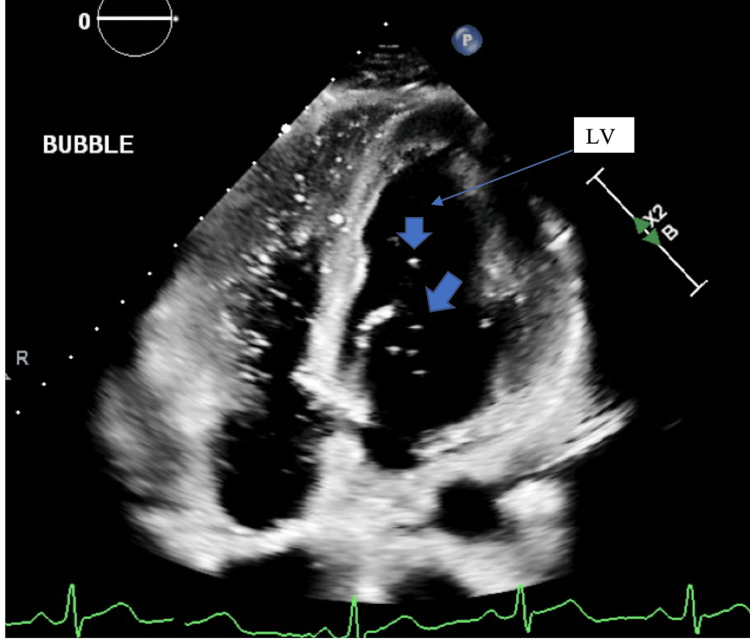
Echocardiogram showing bubbles (arrows) visible in the left ventricle (LV) after the six cardiac cycles
